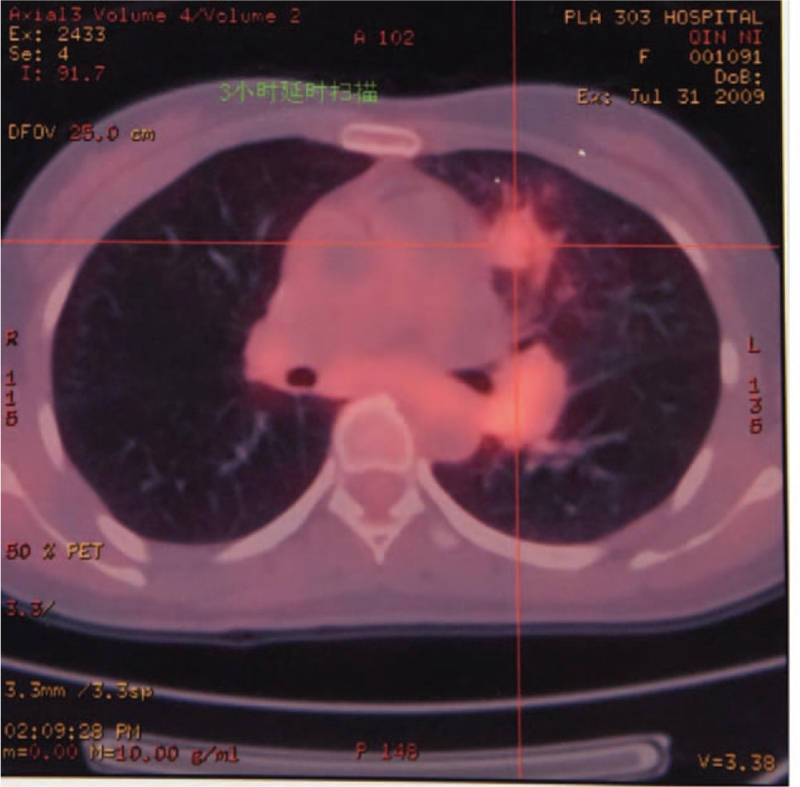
The whole body F18-FDG PET/CT tomography scan (July 31, 2009) showing a pulmonary nodule on upper lobe of the left lung. The radioactive intake is moderate and a nodule on left Lung Gate. F18-FDG-PET/CT = Fluorine 18 fluorodeoxyglucose- Positron emission tomography/computed tomography.
